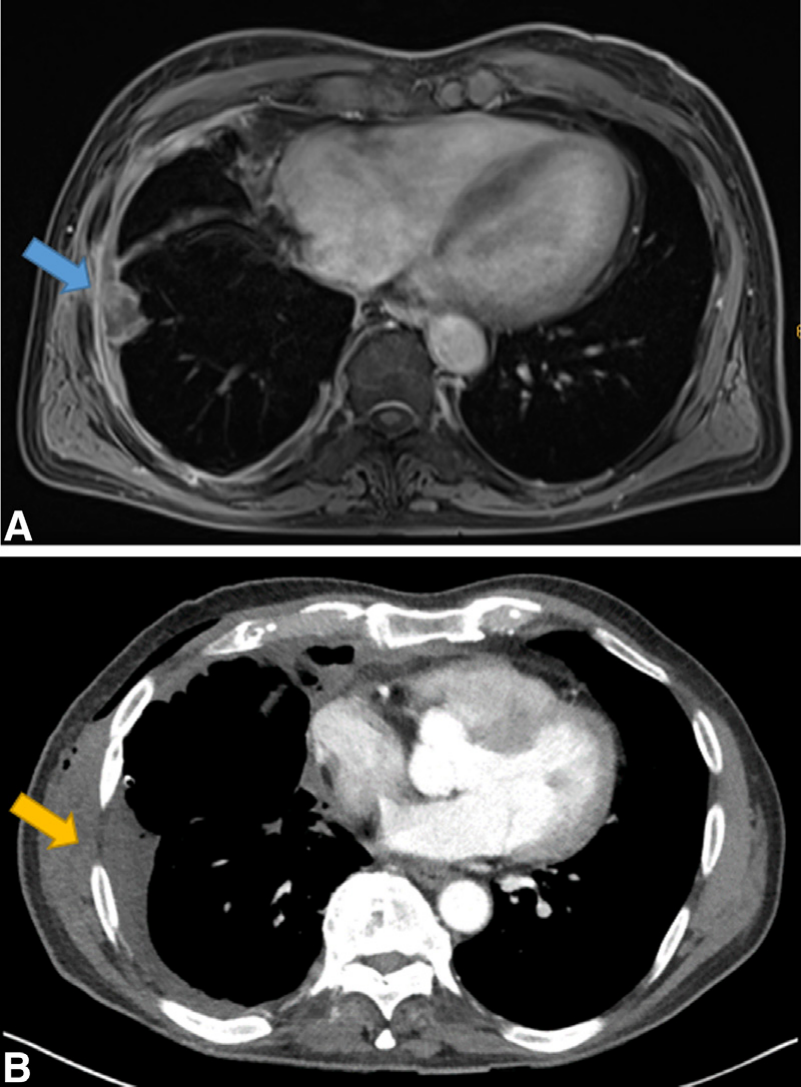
A, Thoracic MRI showing PM chest wall infiltration on the right seventh intercostal space laterally (*blue arrow*). B, Thoracic restaging CT of the same patient without evidence of PM chest wall infiltration (*yellow arrow*). Both *arrows* indicate the intraoperative site of chest wall infiltration in this case. *MRI*, Magnetic resonance imaging; *PM*, pleural mesothelioma; *CT*, computed tomography.
